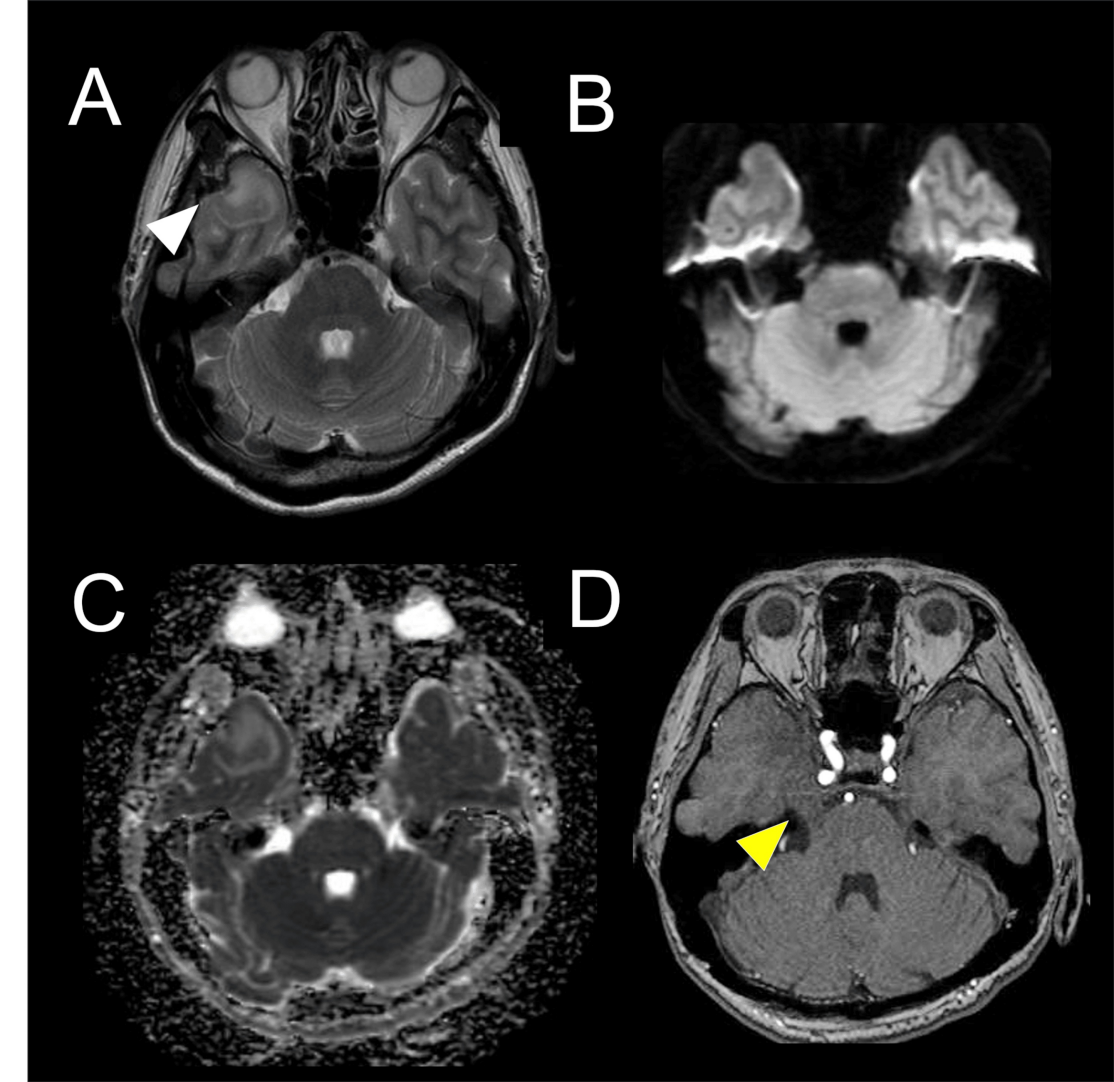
Brain magnetic resonance imaging was performed eight days after onset. T2-weighted imaging revealed a hyperintensity in the white matter of the right temporal lobe (A: white arrowhead), with an iso-intensity on diffusion-weighted imaging (B) and elevated apparent diffusion coefficient values (C). Magnetic resonance angiography revealed an enlarged right trigeminal nerve (D: yellow arrowhead).
